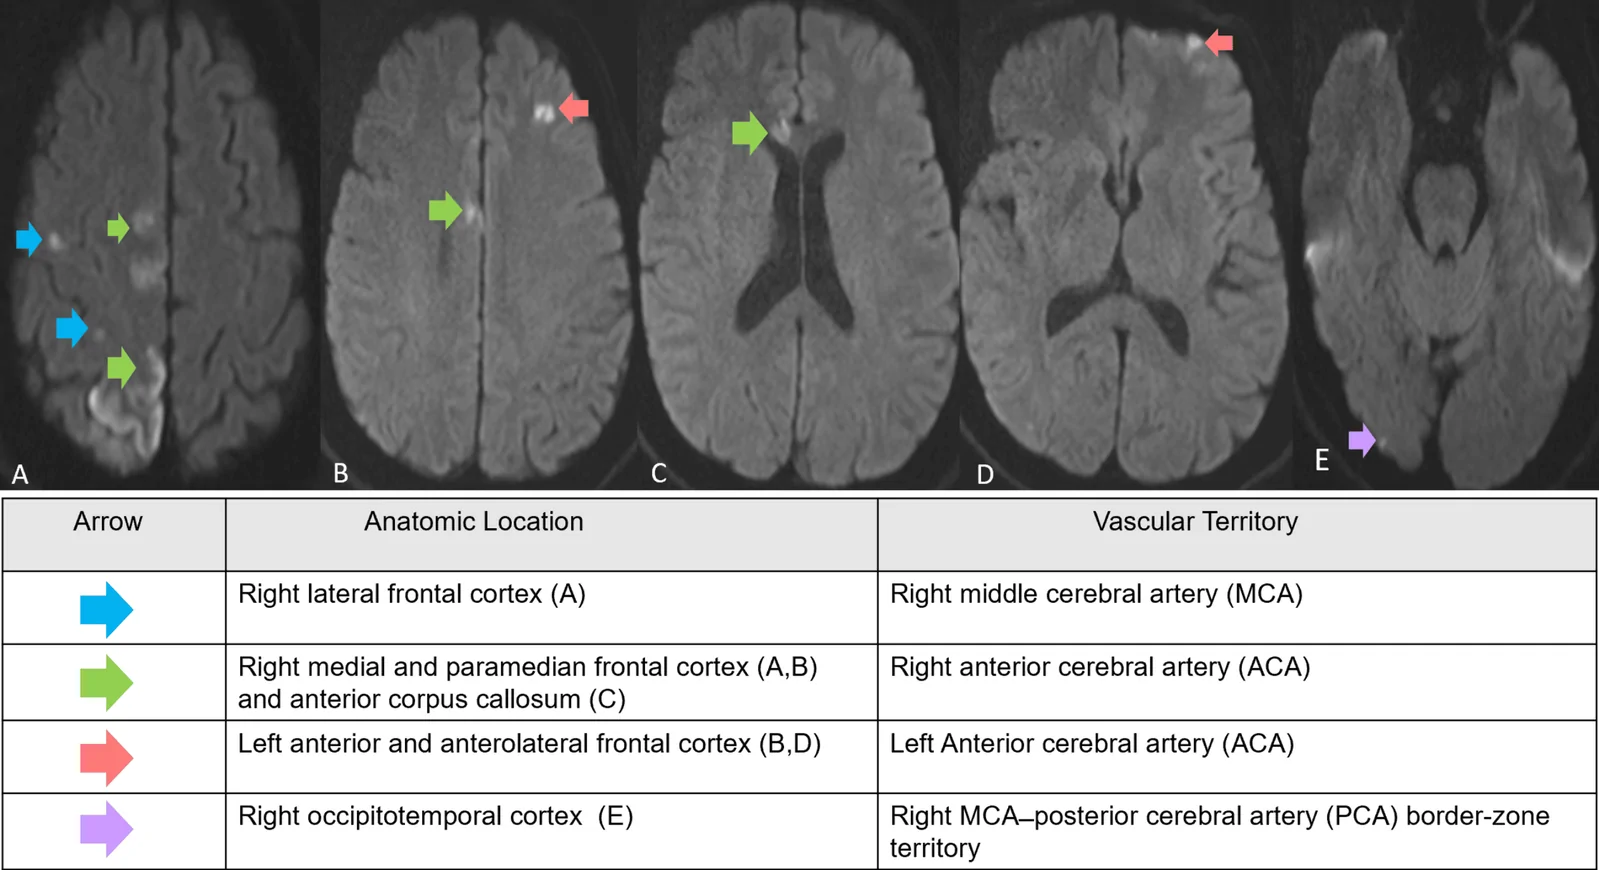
Diffusion-Weighted Magnetic Resonance Imaging Demonstrating Multiterritory Acute Ischemic Infarcts. Diffusion-weighted magnetic resonance imaging demonstrating multiple punctate acute cortical and subcortical infarcts involving the right middle cerebral artery (MCA), right anterior cerebral artery (ACA), and left ACA distributions. Blue arrows indicate right lateral frontal cortical infarcts in the right MCA territory; green arrows indicate right medial/paramedian frontal and anterior corpus callosal infarcts in the right ACA territory; red arrows indicate left frontal infarcts in the distal left ACA distribution, including the ACA-MCA cortical border-zone region; and the purple arrow indicates a right MCA-posterior cerebral artery (PCA) cortical border-zone infarct.
